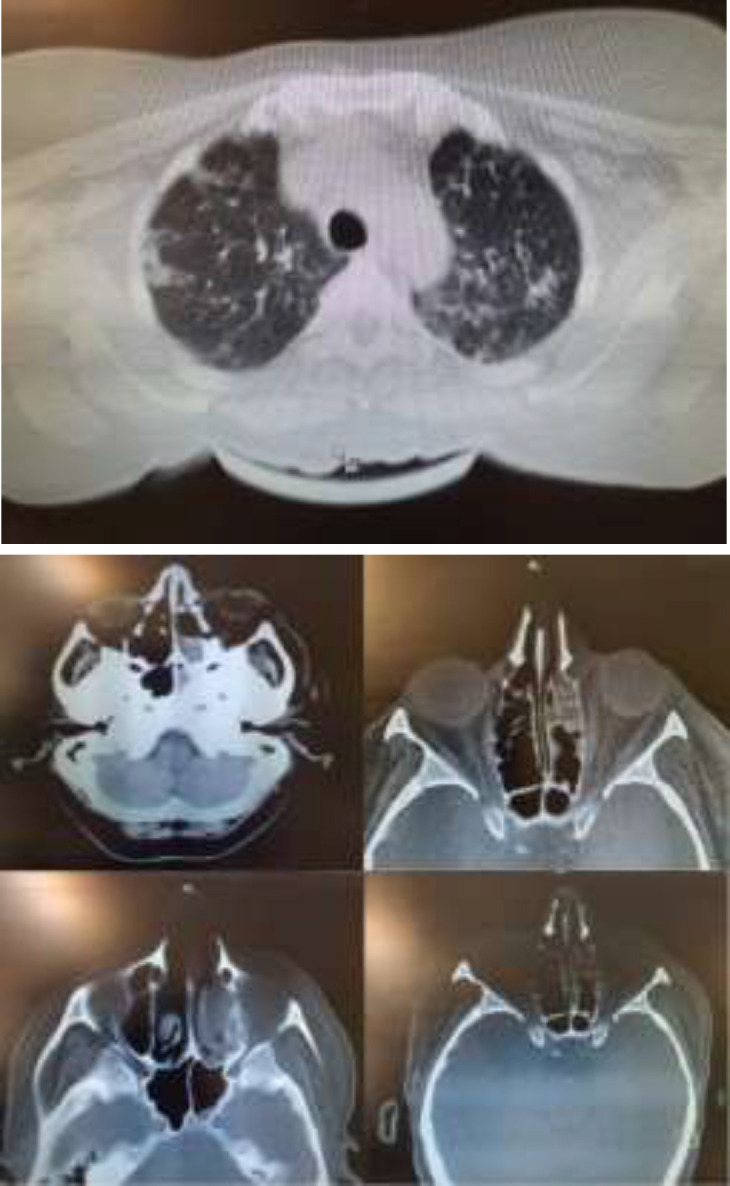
(A): Spiral chest CT scan, (B); Paranasal and periorbital sinus
